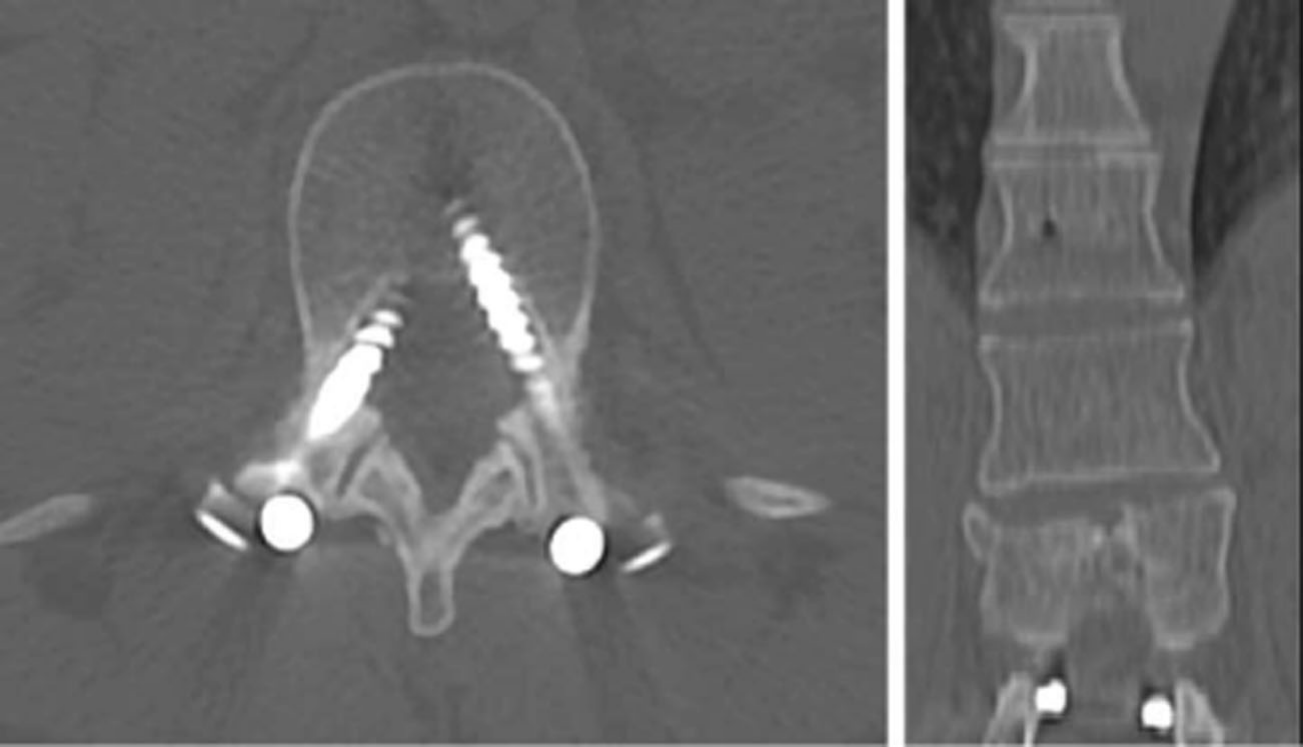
A 31-year-old female: Motor vehicle accident and compression fracture of T12: axial CT (a) and coronal reformats (b) show misplaced bilateral transpedicular screws at L1 abutting the medial cortex of pedicles
